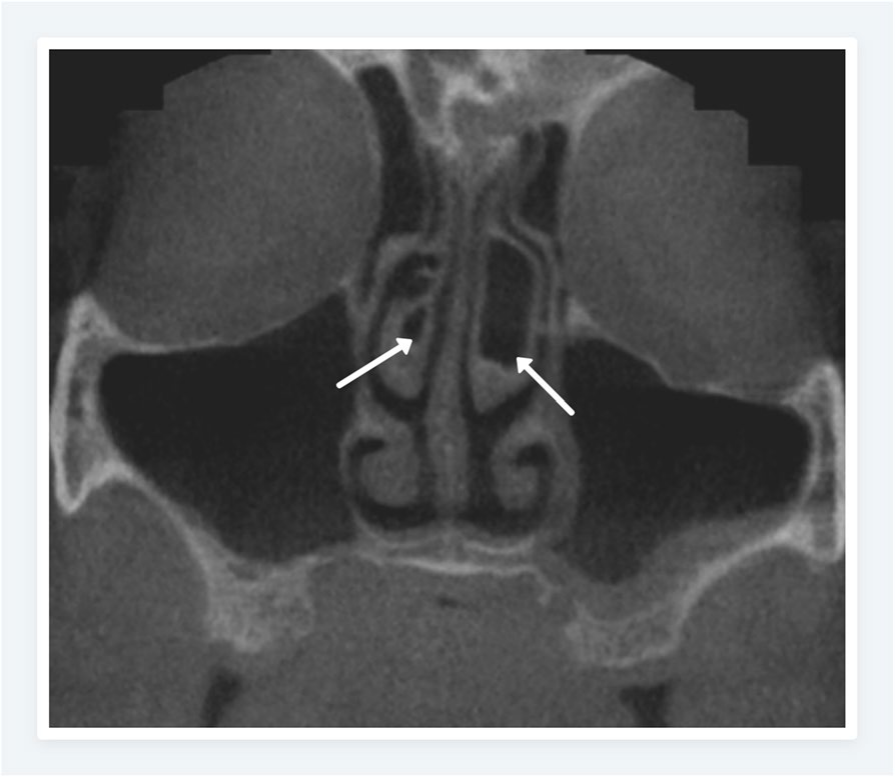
Coronal CBCT image showing the bulbous (right) and extensive (left) type concha bullosa with maxillary sinus mucosal thickening on the left side
